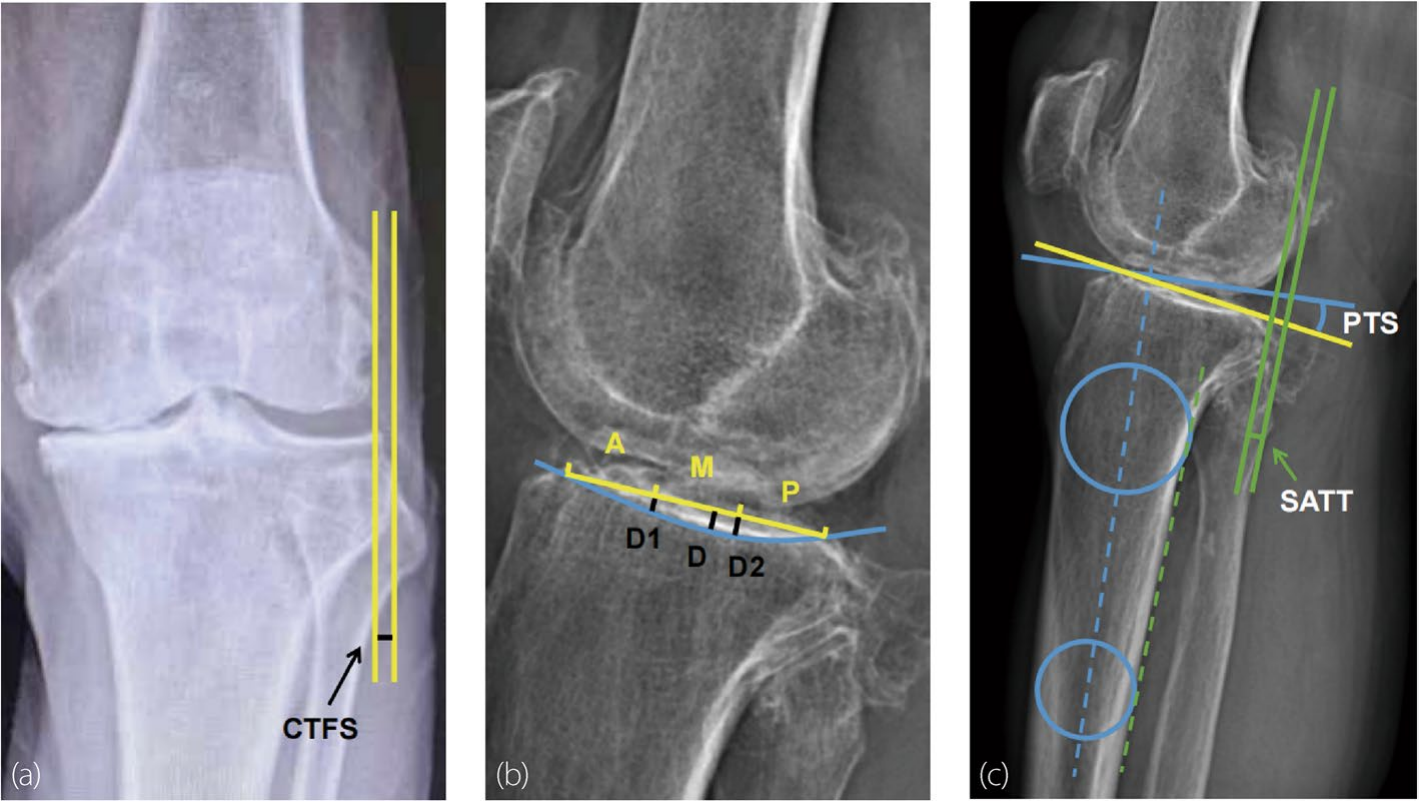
Assessment of radiographic features. a Anteroposterior X-ray. CTFS was defined as the distance between the tangent line (yellow solid line) to the outermost joint edge of the lateral condyle of the femur and the tangent line of the lateral tibial plateau; b Lateral X-ray. Medial tibial plateau was divided into three parts: anterior (A), middle (M) and posterior (P). D1 and D2 were measured at the three equidistant points along the medial tibial plateau. D was the maximum depth of wear on the medial tibial plateau; c Full-length lower limb X-ray. PTS was the angle formed between a line (blue solid line) perpendicular to the tibial diaphyseal axis (blue dotted line) and a line (yellow solid line) tangent to the most superior points at the anterior and posterior edges of the medial plateau. SATT was the distance between two lines (green solid lines) which were tangent to the posterior part of the medial plateau and medial femoral condyle, paralleling to the posterior tibial cortex (green dotted line)
